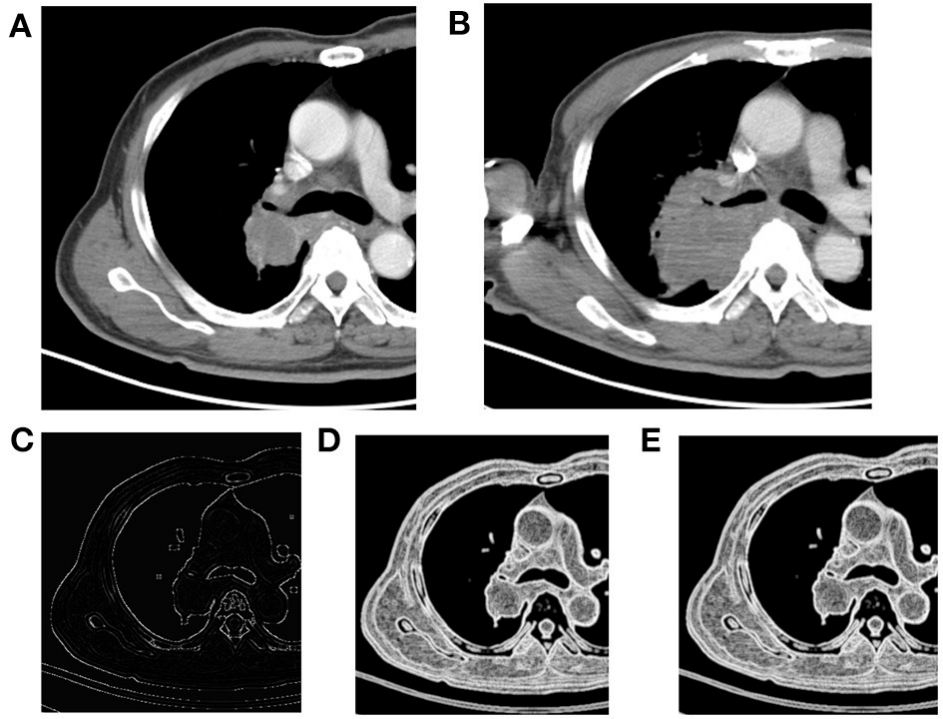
Right upper lobe mass, NSCLC. (A) Pre-treatment contrast-enhanced CT; (B) contrast-enhanced CT 8 weeks later after treatment, the efficacy evaluation was progression (PD); (C) kurtosis map; (D) S(1,0) SumEntrp map; (E) S(2,2) SumEntrp map.
